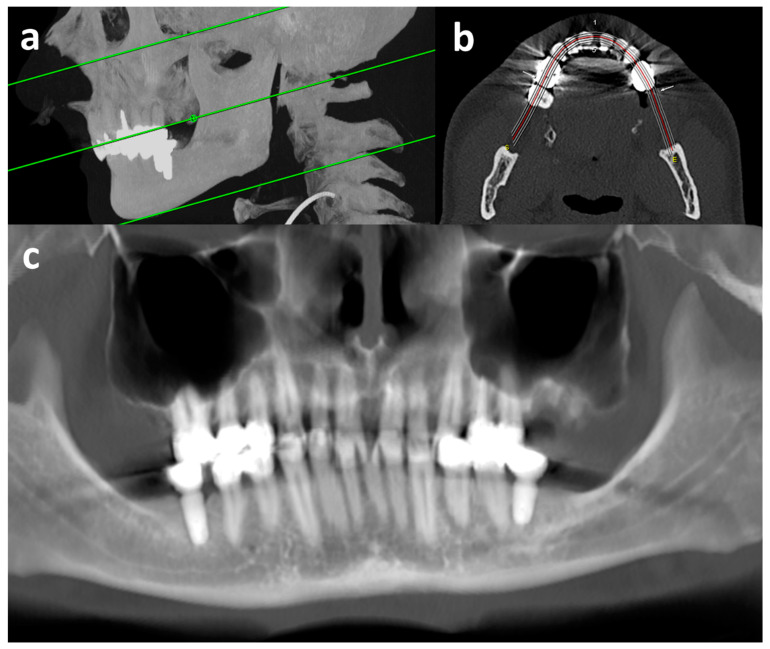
Reconstruction of vOPG from CT. Sagittal maximum intensity projection (a) for level selection of upper and lower limits and occlusal plain (green lines), para-axial plain (b) for contour selection of alveolar processes (red line: center of reconstruction), and margin selection of maximum intensity projection for the vOPG (outer white lines), and final vOPG (c) with very good image quality; CT = computed tomography examination; vOPG = virtual orthopantomogram.
